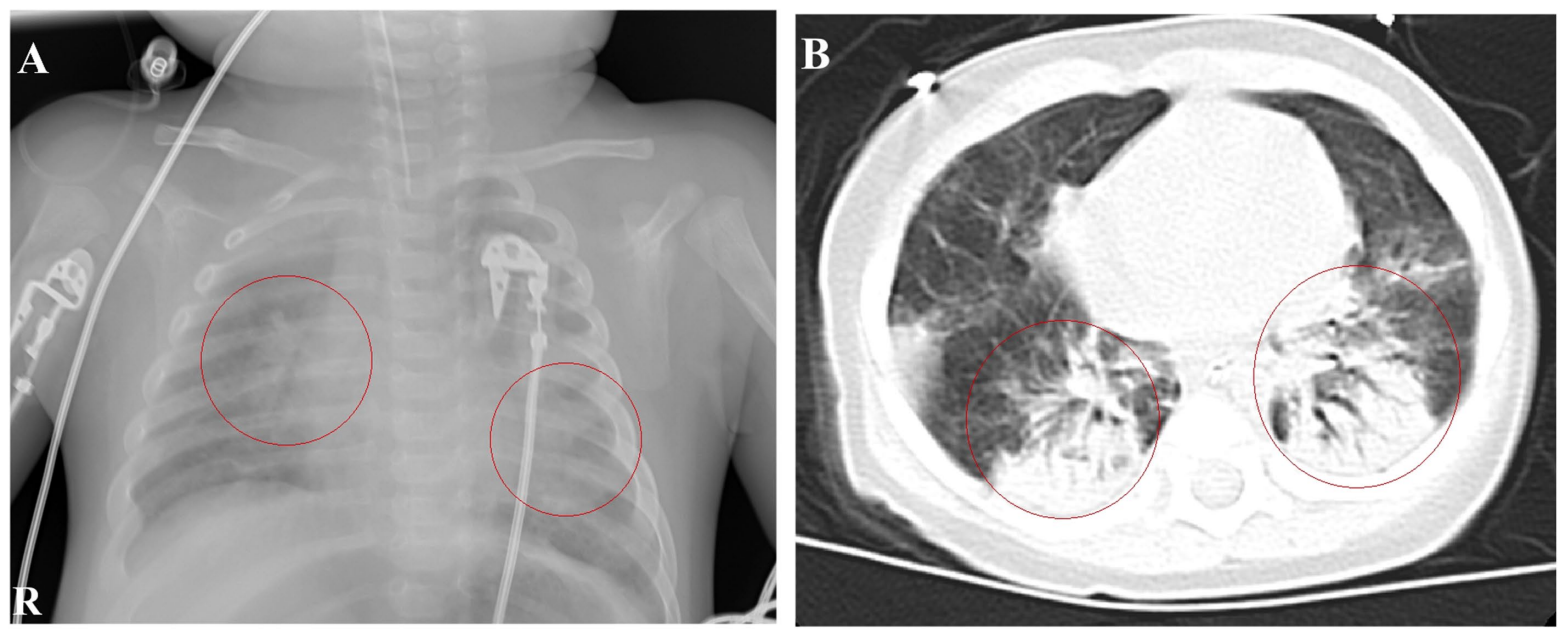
Chest imaging at admission. (A) Chest radiograph on January 9th shows patchy shadows in both lungs. (B) Chest CT on January 10th reveals multiple patchy hyperdense and solid shadows.
